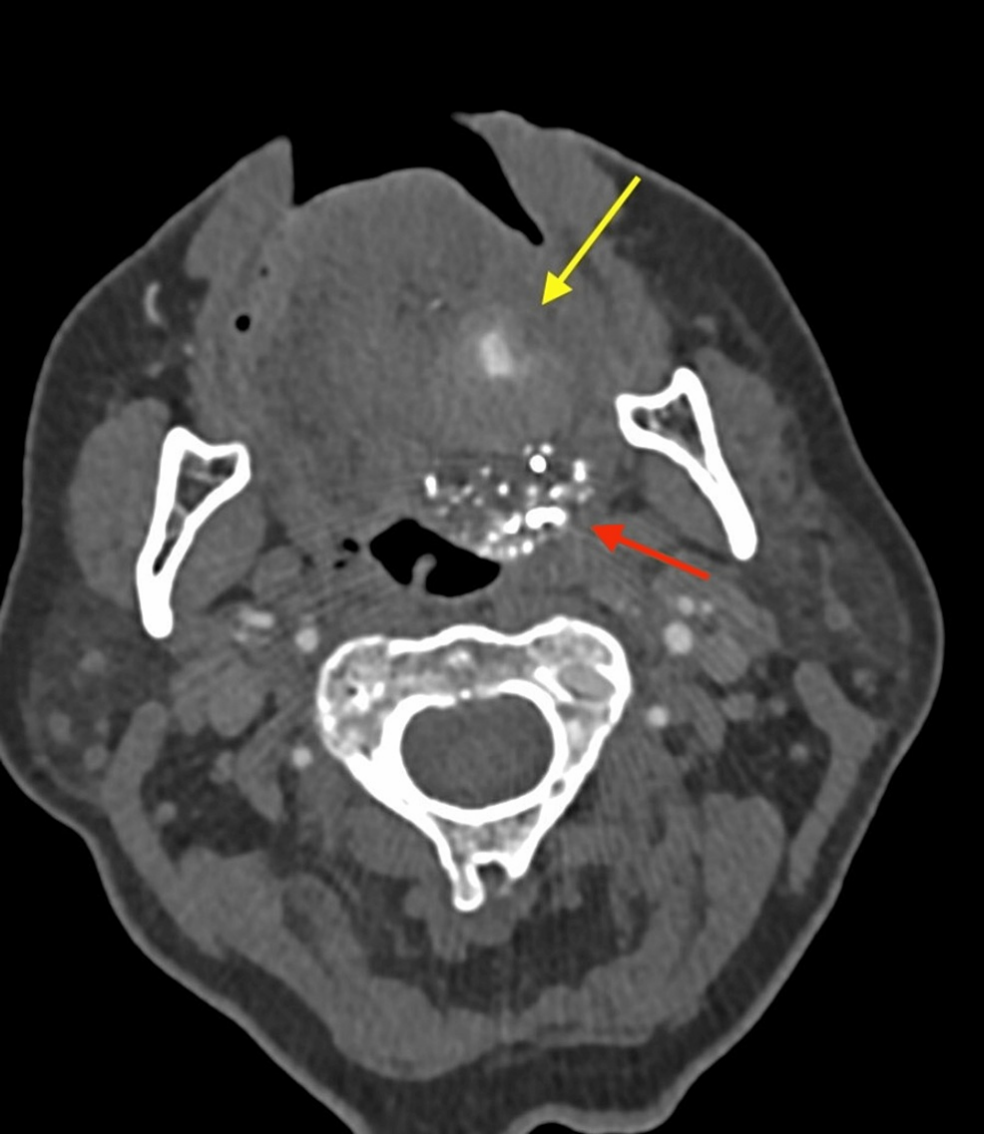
Axial computed tomographic angiography image one-month following endovascular embolization demonstrating a small, ovoid lesion with a residual surrounding hematoma outline (yellow arrow) concerning for potentially residual pseudoaneurysm. Hyperdensity present posterior to the lesion (red arrow) is the embolization material.
